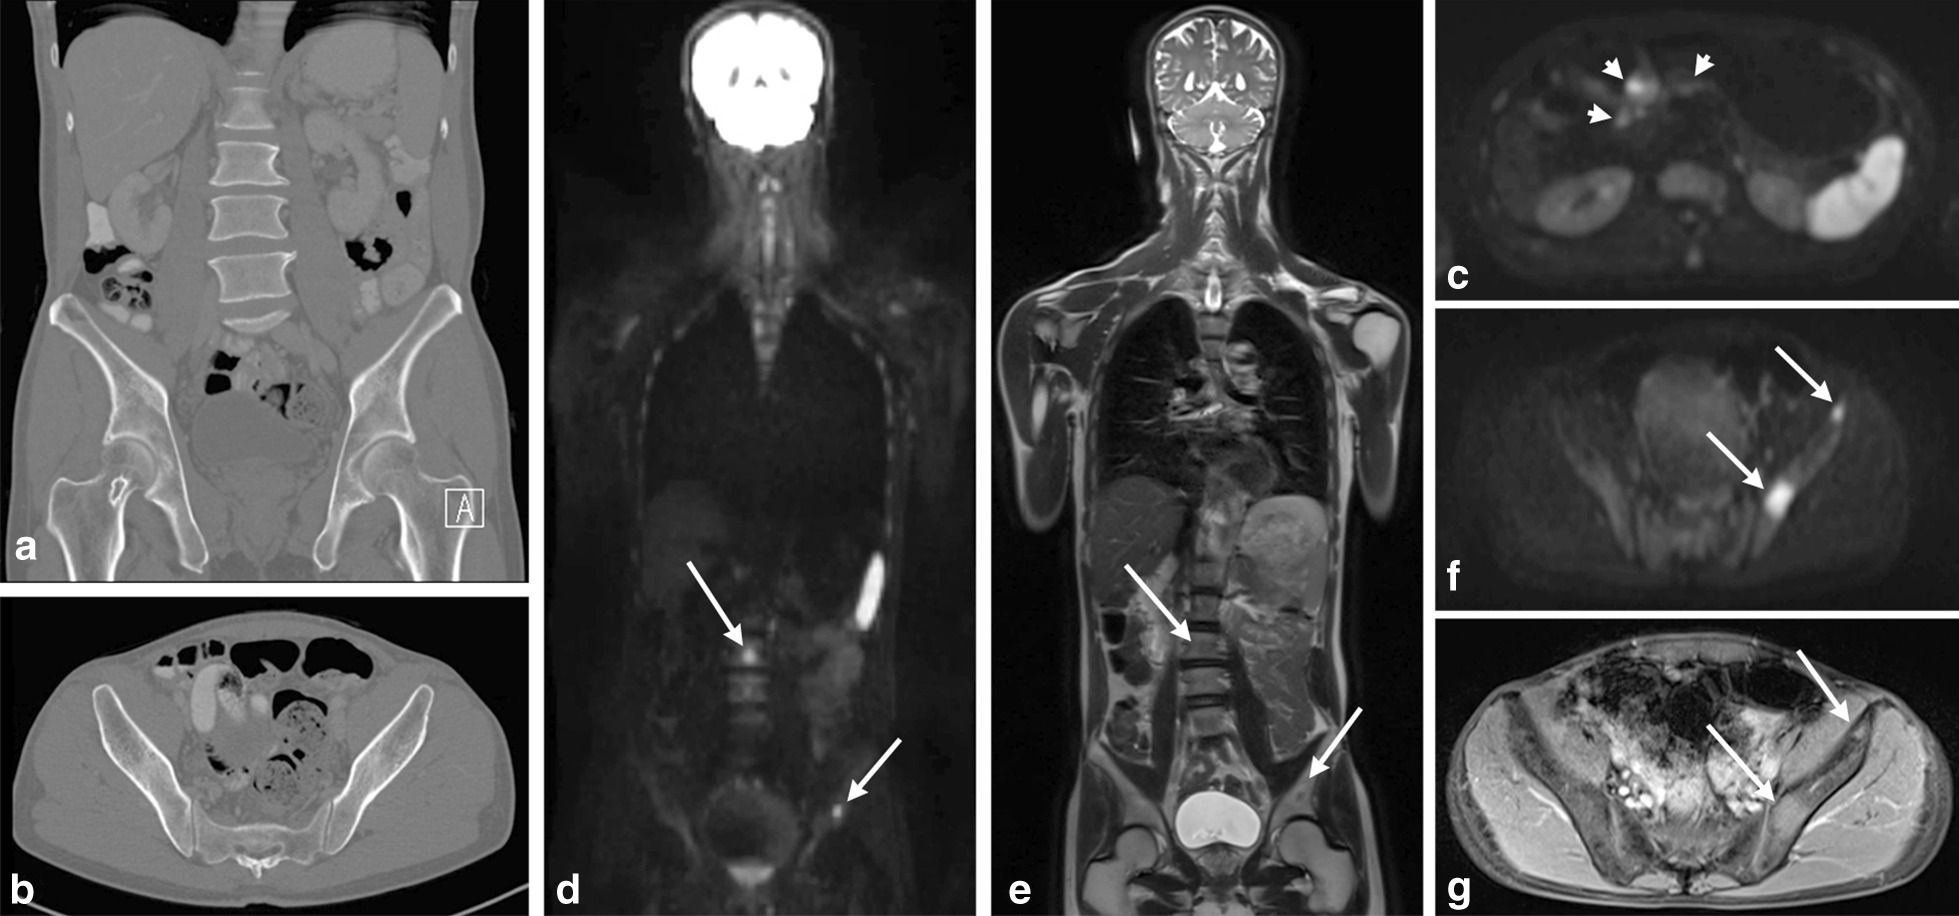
A patient with an age-range between 60 and 70 years old with a primary gastric cancer, where CT did not show any distant metastases (a, b), underwent a WB-DWI/MRI for operability assessment. Axial b1000 DWI images revealed millimetric tumor implants on the pancreatic surface (c, arrowheads). At the same time, multiple bone metastases (arrows) could be seen on the coronal b1000 DWI (d), coronal T2-weighted images (e), axial b1000 DWI (f) and post-contrast T1-weighted images (g)
